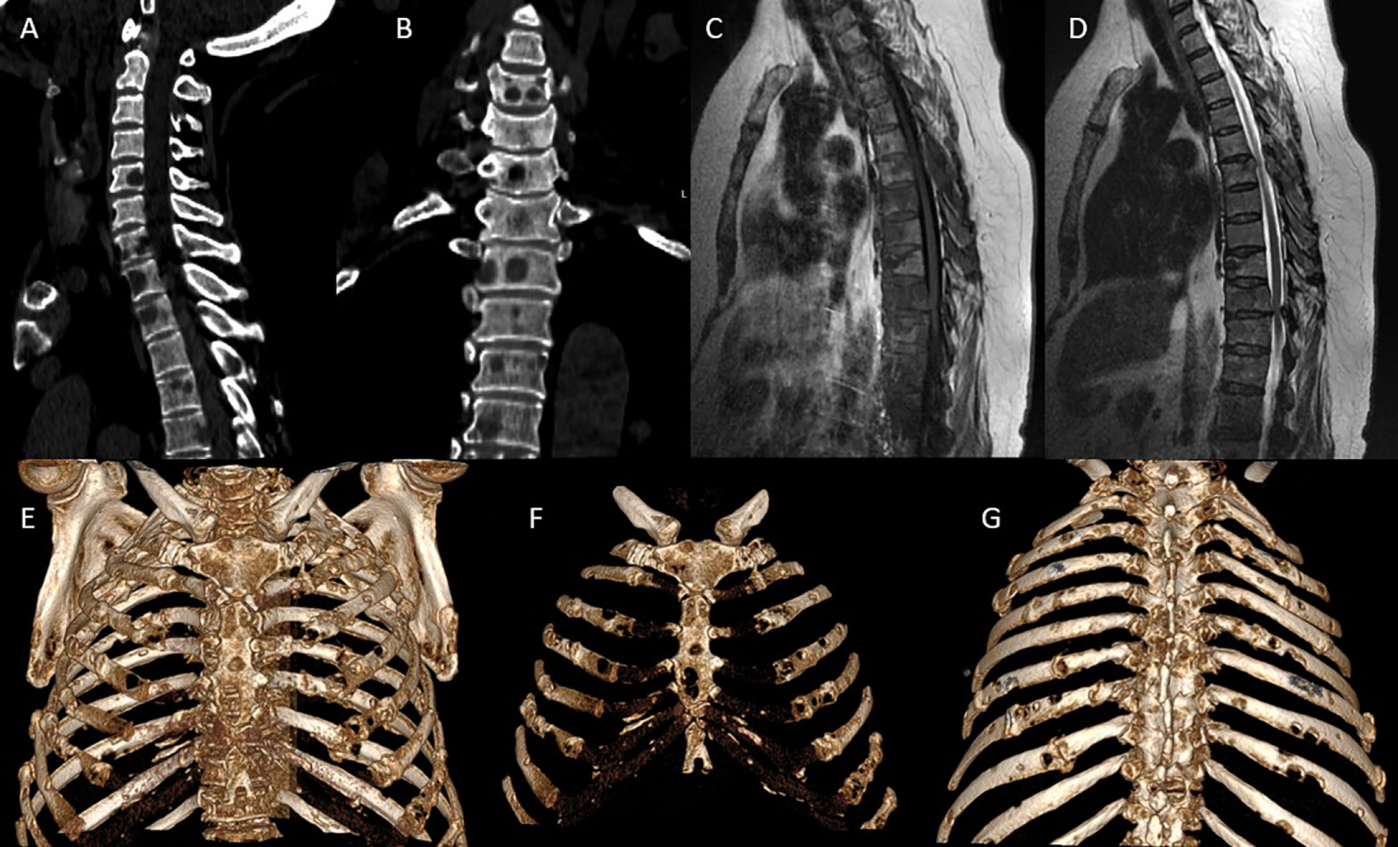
Preoperative CT/MRI imaging: (A, B) Multislice CT: sagittal (A) and frontal (B) reconstructions show multiple osteolytic lesions in the vertebral bodies from Th1 to Th12, with vertebral body collapse primarily at Th6-Th10, reduced intervertebral disc height, cortical bone destruction, and involvement of the posterior vertebral elements including the arches and facet joints. Extensive vertebral destruction is accompanied by lytic changes in the ribs and sternum. (C, D) Sagittal thoracic spine MRI: on T1-weighted images (C), hypointense metastatic lesions are seen replacing the bone marrow of thoracic vertebrae. On T2-weighted images (D), a distinct posterior paravertebral soft tissue component is evident, with spinal cord compression at Th10-Th11, associated with dural sac deformation and spinal canal stenosis. (E–G) 3D CT reconstructions of the thorax in various projections: anterior (E), frontal (F), and posterior (G) views demonstrate multiple metastatic rib lesions with lytic destruction, predominantly along the posterolateral arcs, as well as involvement of the sternum, including both the manubrium and body. Notable findings include thoracic cage deformation and signs of pathological fractures with resulting bone defects.
